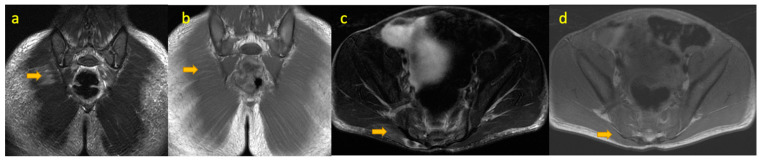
(a–d)—Haemophagocytic lymphohistiocytosis in an 8-year-old boy who initially presented with 2 weeks’ history of painful right-sided limp and raised CRP. MRI of the pelvis (coronal T1W, STIR, axial T1W, and T2WFS images) reveals focal nonspecific muscle oedema signal and mild enlargement of the right gluteus maximus muscle.
